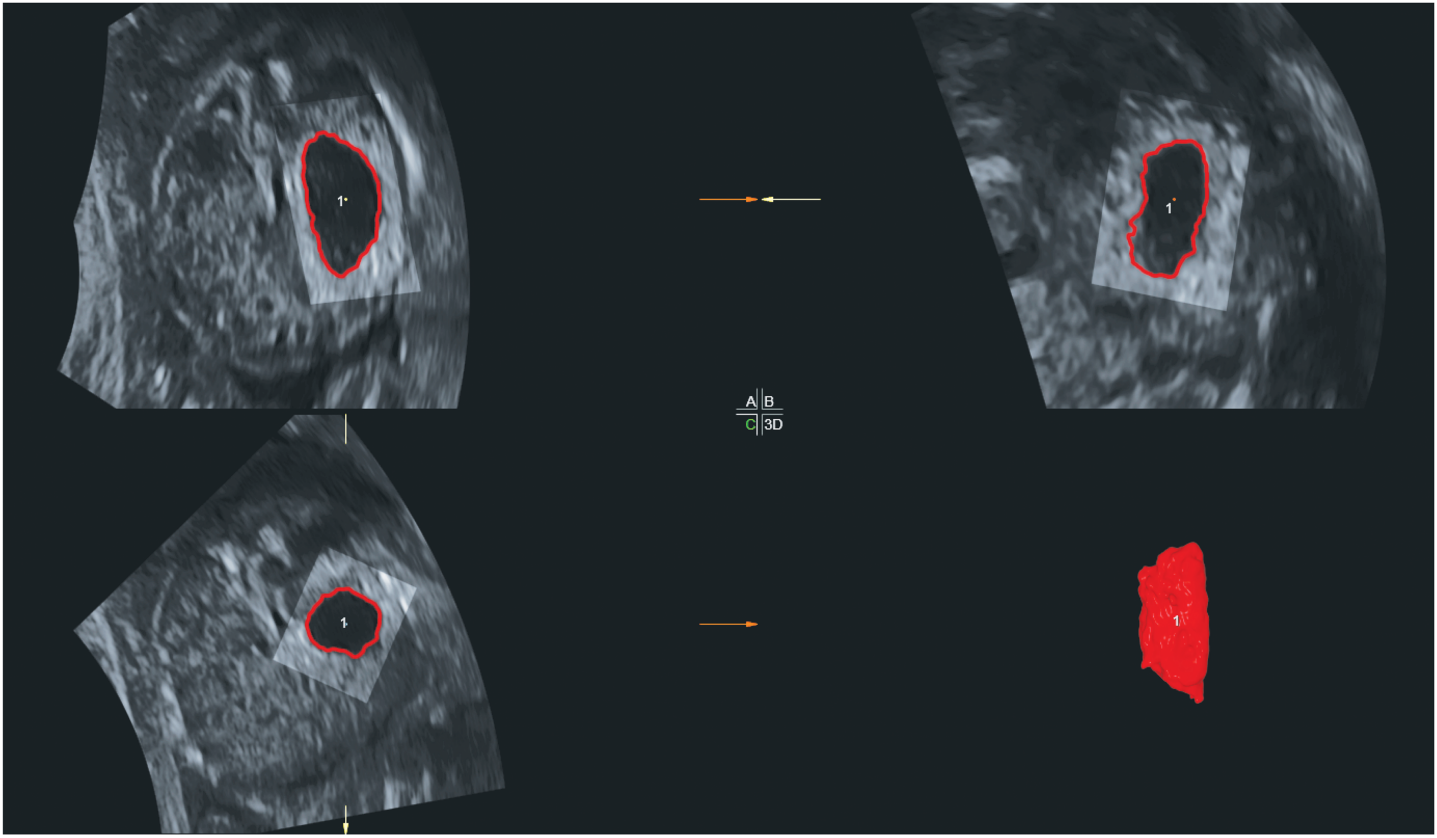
Fetal stomach volume at 21 weeks' gestation, measured using Sonography‐based Automated Volume Count (SonoAVC) [Colour figure can be viewed at http://wileyonlinelibrary.com]
